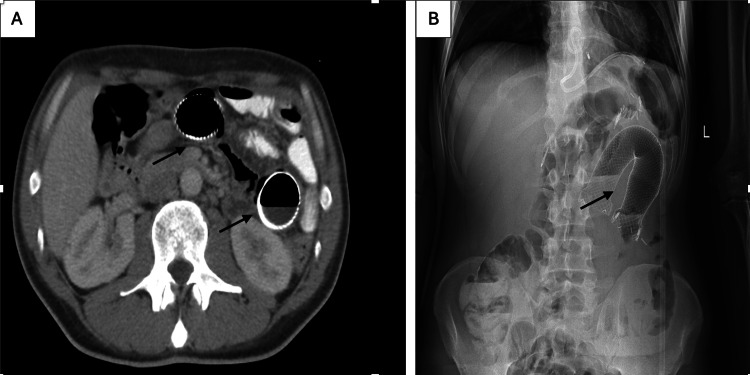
(A) Erect abdominal X-ray showing a self-expandable metal stent (SEMS) in the left upper quadrant of the abdomen. (B) Abdominal CT scan showing a migrated 36 mm SEMS in the proximal jejunum, associated with bowel dilation.
